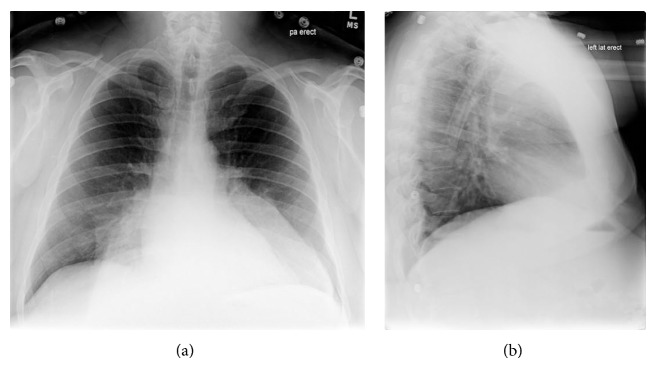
The PA (a) radiograph of the chest reveals a double-density opacity overlying the cardiac silhouette which on lateral (b) radiograph does not appear to localize to the anterior thorax or any compartment of the mediastinum.
